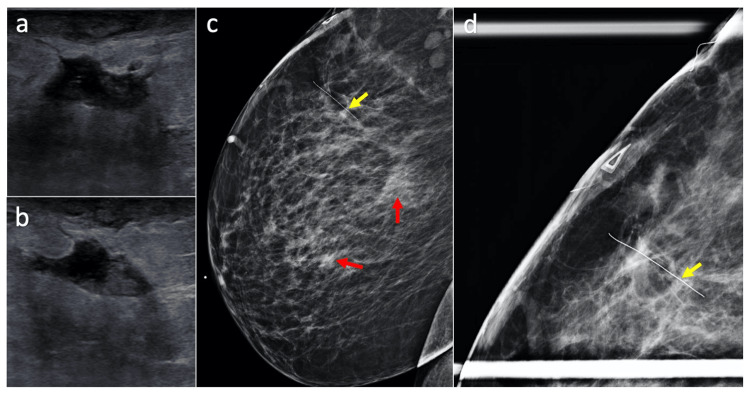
Imaging of breast abscess Images A and B are perpendicular ultrasound views of an 18 × 17 × 8 mm^3^ hypoechoic mass in the right breast at 11 o'clock, 13 cm from the nipple at an area of palpable concern. Signs of mild skin thickening and trabecular thickening are present. Images C and D show tomosynthesis imaging of the right breast with a mediolateral view and signs of scattered fibroglandular density present (red arrows). Image d includes compression of the area of palpable concern demarcated by the wire (yellow arrows).
